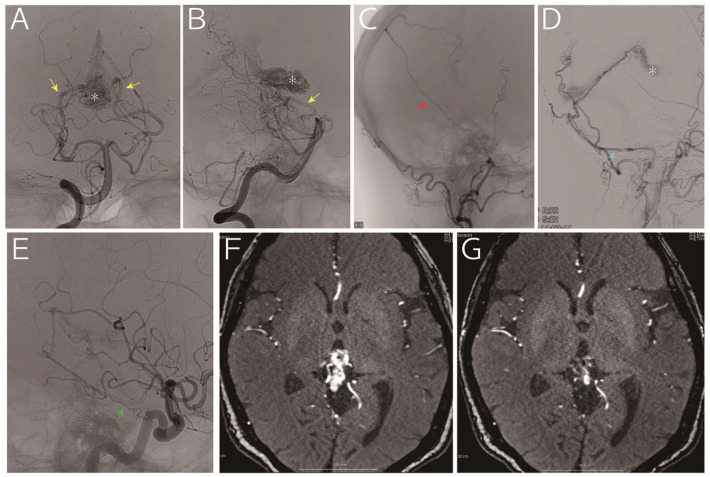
A 63-year-old woman who presented with headache and vertigo without any neurological deficits was referred to us for treatment of an arteriovenous malformation. Digital subtraction angiography (A and B, vertebral artery; C: right external carotid artery; D, left external carotid artery; and E, left internal carotid artery) indicated a nidus (asterisk) fed by the medial posterior choroidal artery (yellow arrow), posterior cerebral artery perforators, middle meningeal artery (red arrow), posterior meningeal artery (light blue arrow, via the occipital artery) and tentorial artery (green arrow). The drainage vein was the vein of Galen. Considering that a part of the nidus was embedded in the thalamus (F, time-of-flight magnetic resonance imaging), we decided to treat using gamma knife, and the images obtained 30 months later showed remarkable reduction in the nidus without any sings of hemorrhage (G, time-of-flight magnetic resonance imaging).
